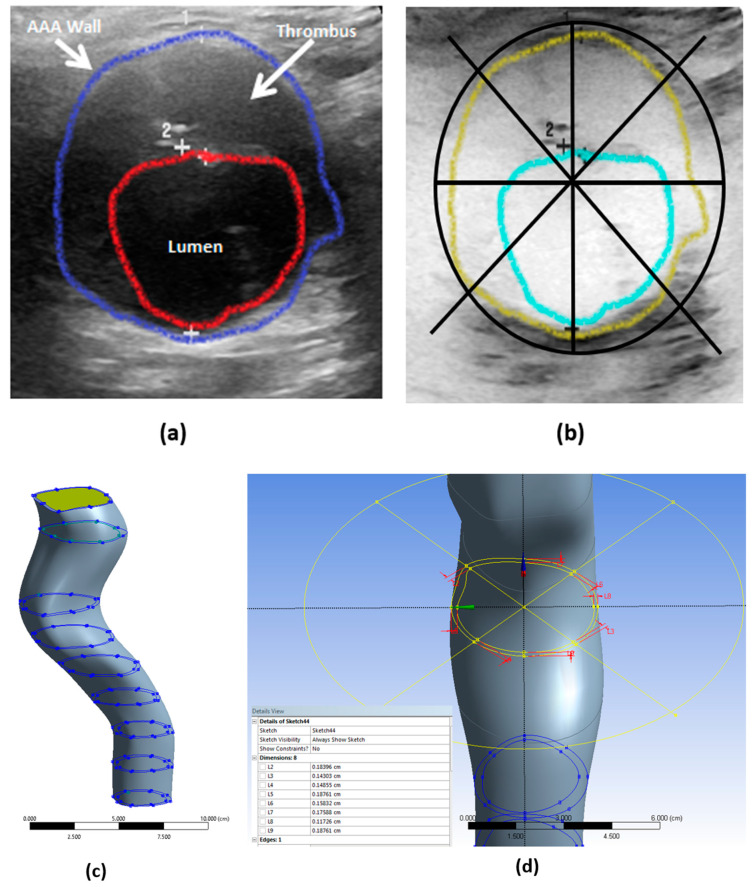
Patient-Specific Aneurysm Rupture Prediction (P-SARP) method. (a) Dimensions using ultrasound-segmented images template. (b) Arterial wall thickness measurements at a 45° angle, (c) Conversion of the 2D segments to 3D STL geometry, and (d) the dimensions to create each segment.
